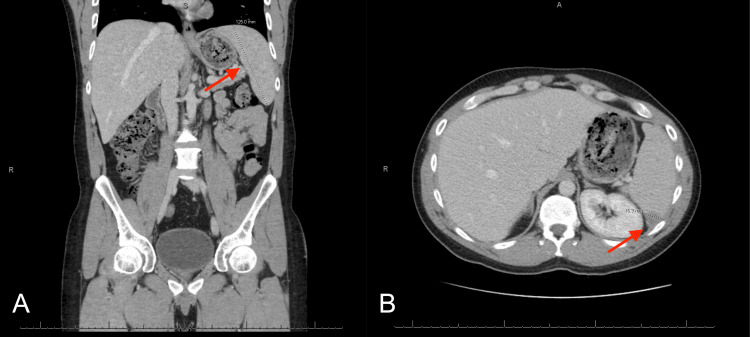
CT abdomen and pelvis with IV contrast (A) Splenomegaly measuring 12.5 cm. (B) Lesion in the posterior aspect of the spleen measuring up to 16.9 mm.
